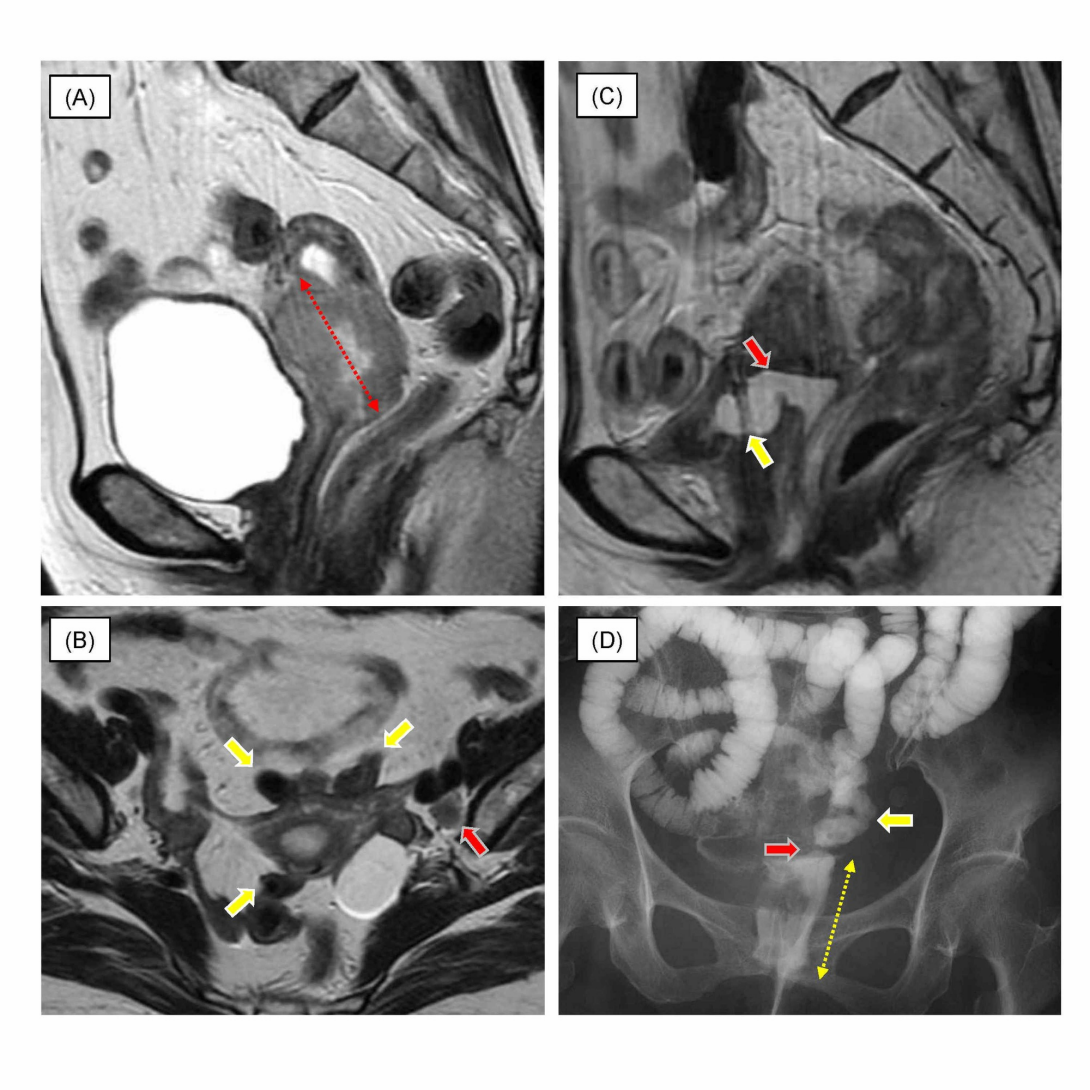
Magnetic resonance imaging (MRI) of the pelvis and radiographic enteroclysis. T2-weighted MRI of the pelvis before radiotherapy (RT) (A, B), and at two years and five months after RT (C). Radiographic enteroclysis with gastrografin at seven years and two months after RT (D). Primary tumor (A, arrow). Swelling of the left internal iliac node (B, red arrow) and the small bowel around the uterus (B, yellow arrow). Site of the fistula between the vagina and bladder (C, red arrow), and the bladder (C, yellow arrow). Site of the fistula between the vagina and small bowel (D, red arrow), and the small bowl (D, yellow arrow) and vagina (D, yellow dotted arrow).
